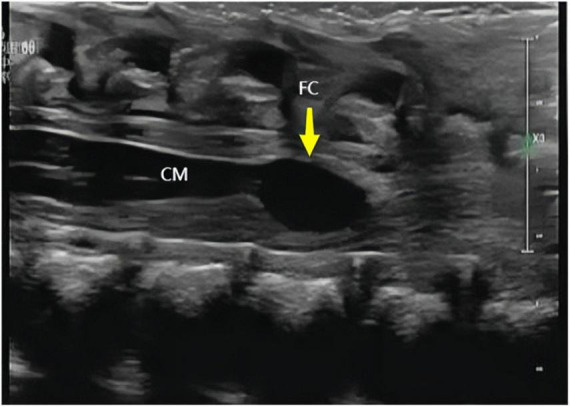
Longitudinal section of the spine obtained using neonatal high-frequency ultrasound. Yellow arrows indicate cysts of the terminal filament (FC) and conus medullaris (CM).
